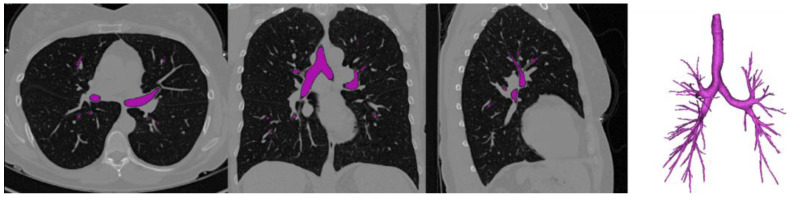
Axial, coronal, and sagittal views of a CT scan from a healthy volunteer. The airways have been segmented using a 3D confidence-connected region-growing algorithm. The original CT data are from [82], acquired at Washington University in St. Louis, MO, USA.
